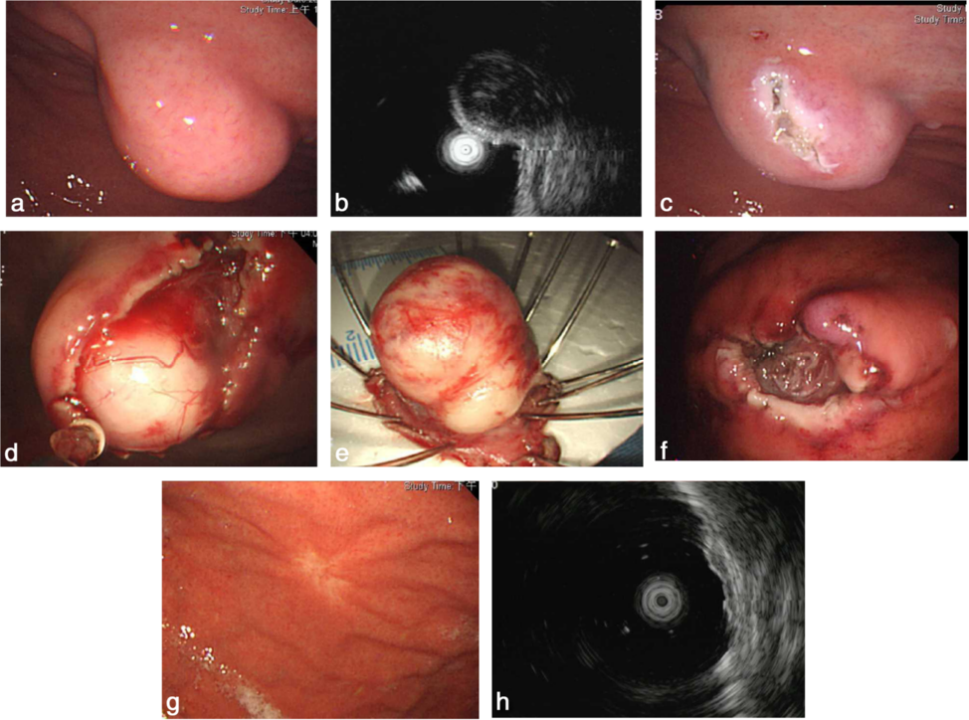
A gastric subepithelial tumor originating from the muscularis propria layer was resected by the modified ESD with enucleation.a. Endoscopic view of the gastric subepithelial tumor at gastric high body. b. Endoscopic ultrasonography (EUS) revealed an inhomogeneous hypoechoic tumor arising from the muscularis propria layer. c. A precut and longitudinal incision of the mucosa. d. Lateral dissection with an insulated-tip knife 2 exposing an encapsulated tumor. e. A slight dumbbell-shaped tumor was enucleated. f. No visible residual tumor at ulcer base. g. A whitish scar was shown 2 months after dissection. h. EUS revealed mild thickening of the second layer and no recurrent tumor in the muscularis propria layer.
